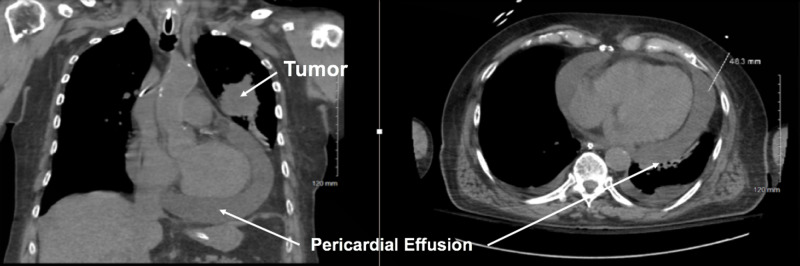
chest CT showing large pericardial effusion and a mass in the upper left lung
